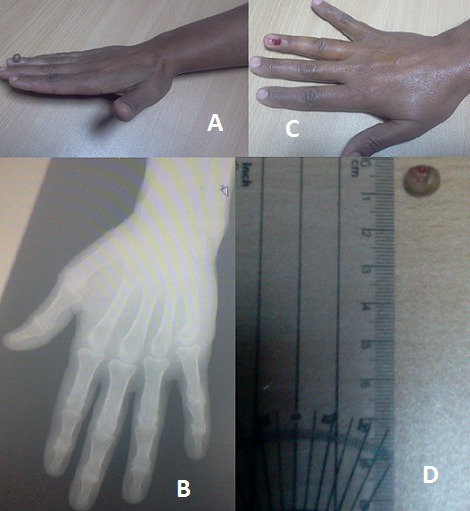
(A): image clinique: nodule mou pédiculé, couleur brune, unguéal au niveau 4ème doigt de la main droite; (B): radiographie main droite: normale; (C): image clinique après résection chirurgicale; (D): image de la tumeur réséquée envoyée pour étude anatomopathologique
